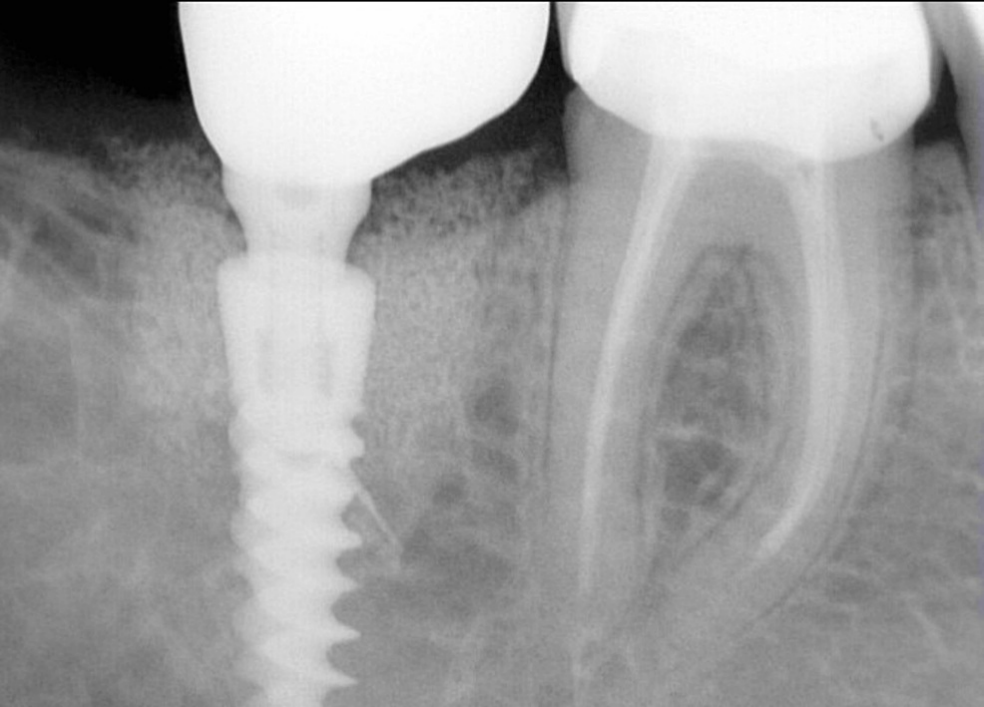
Periapical X-ray showing the implant with the definitive restoration.
